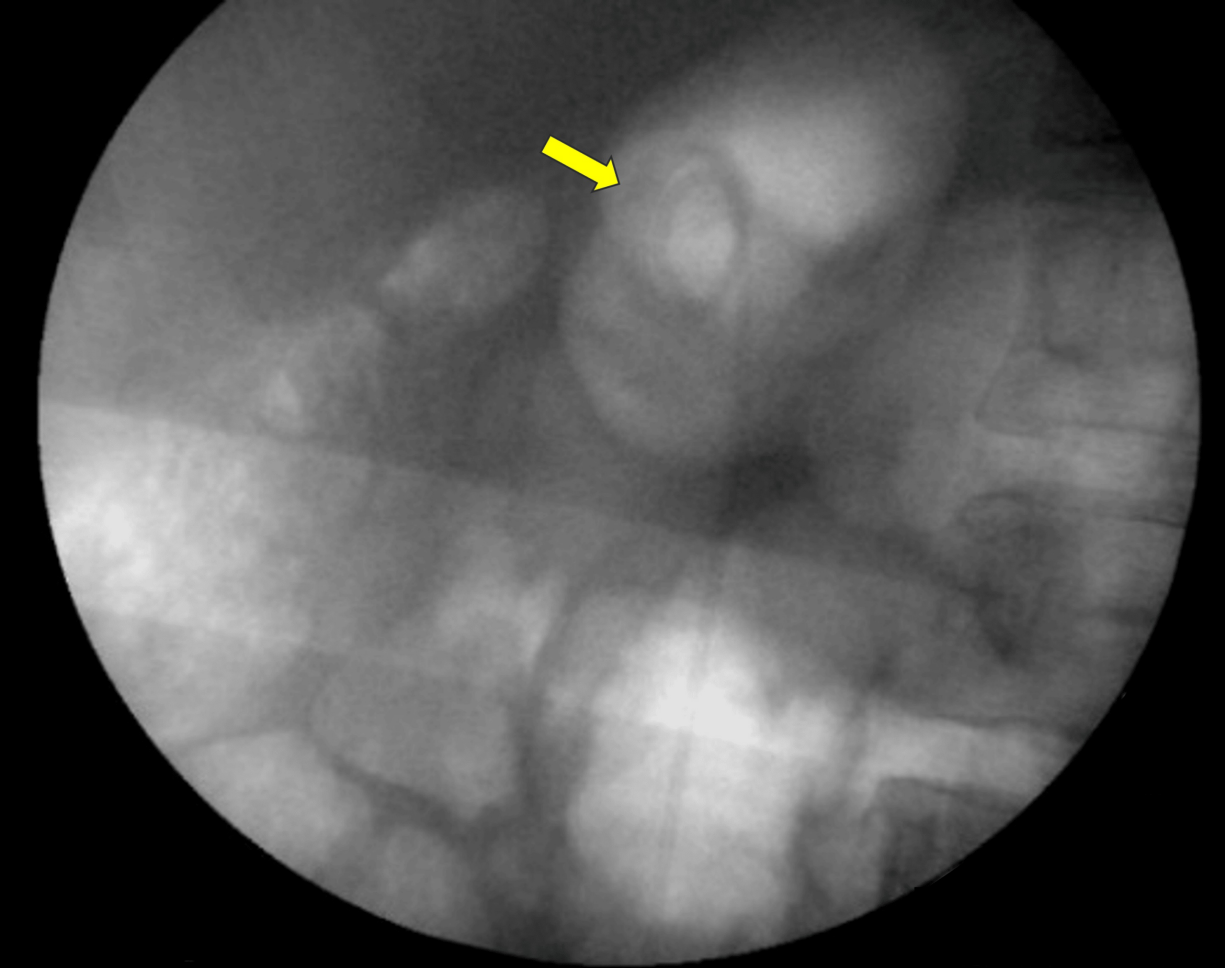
Intraoperative X-ray confirming the complete removal of all components of the retained JJ stent (arrow). JJ, double-J
